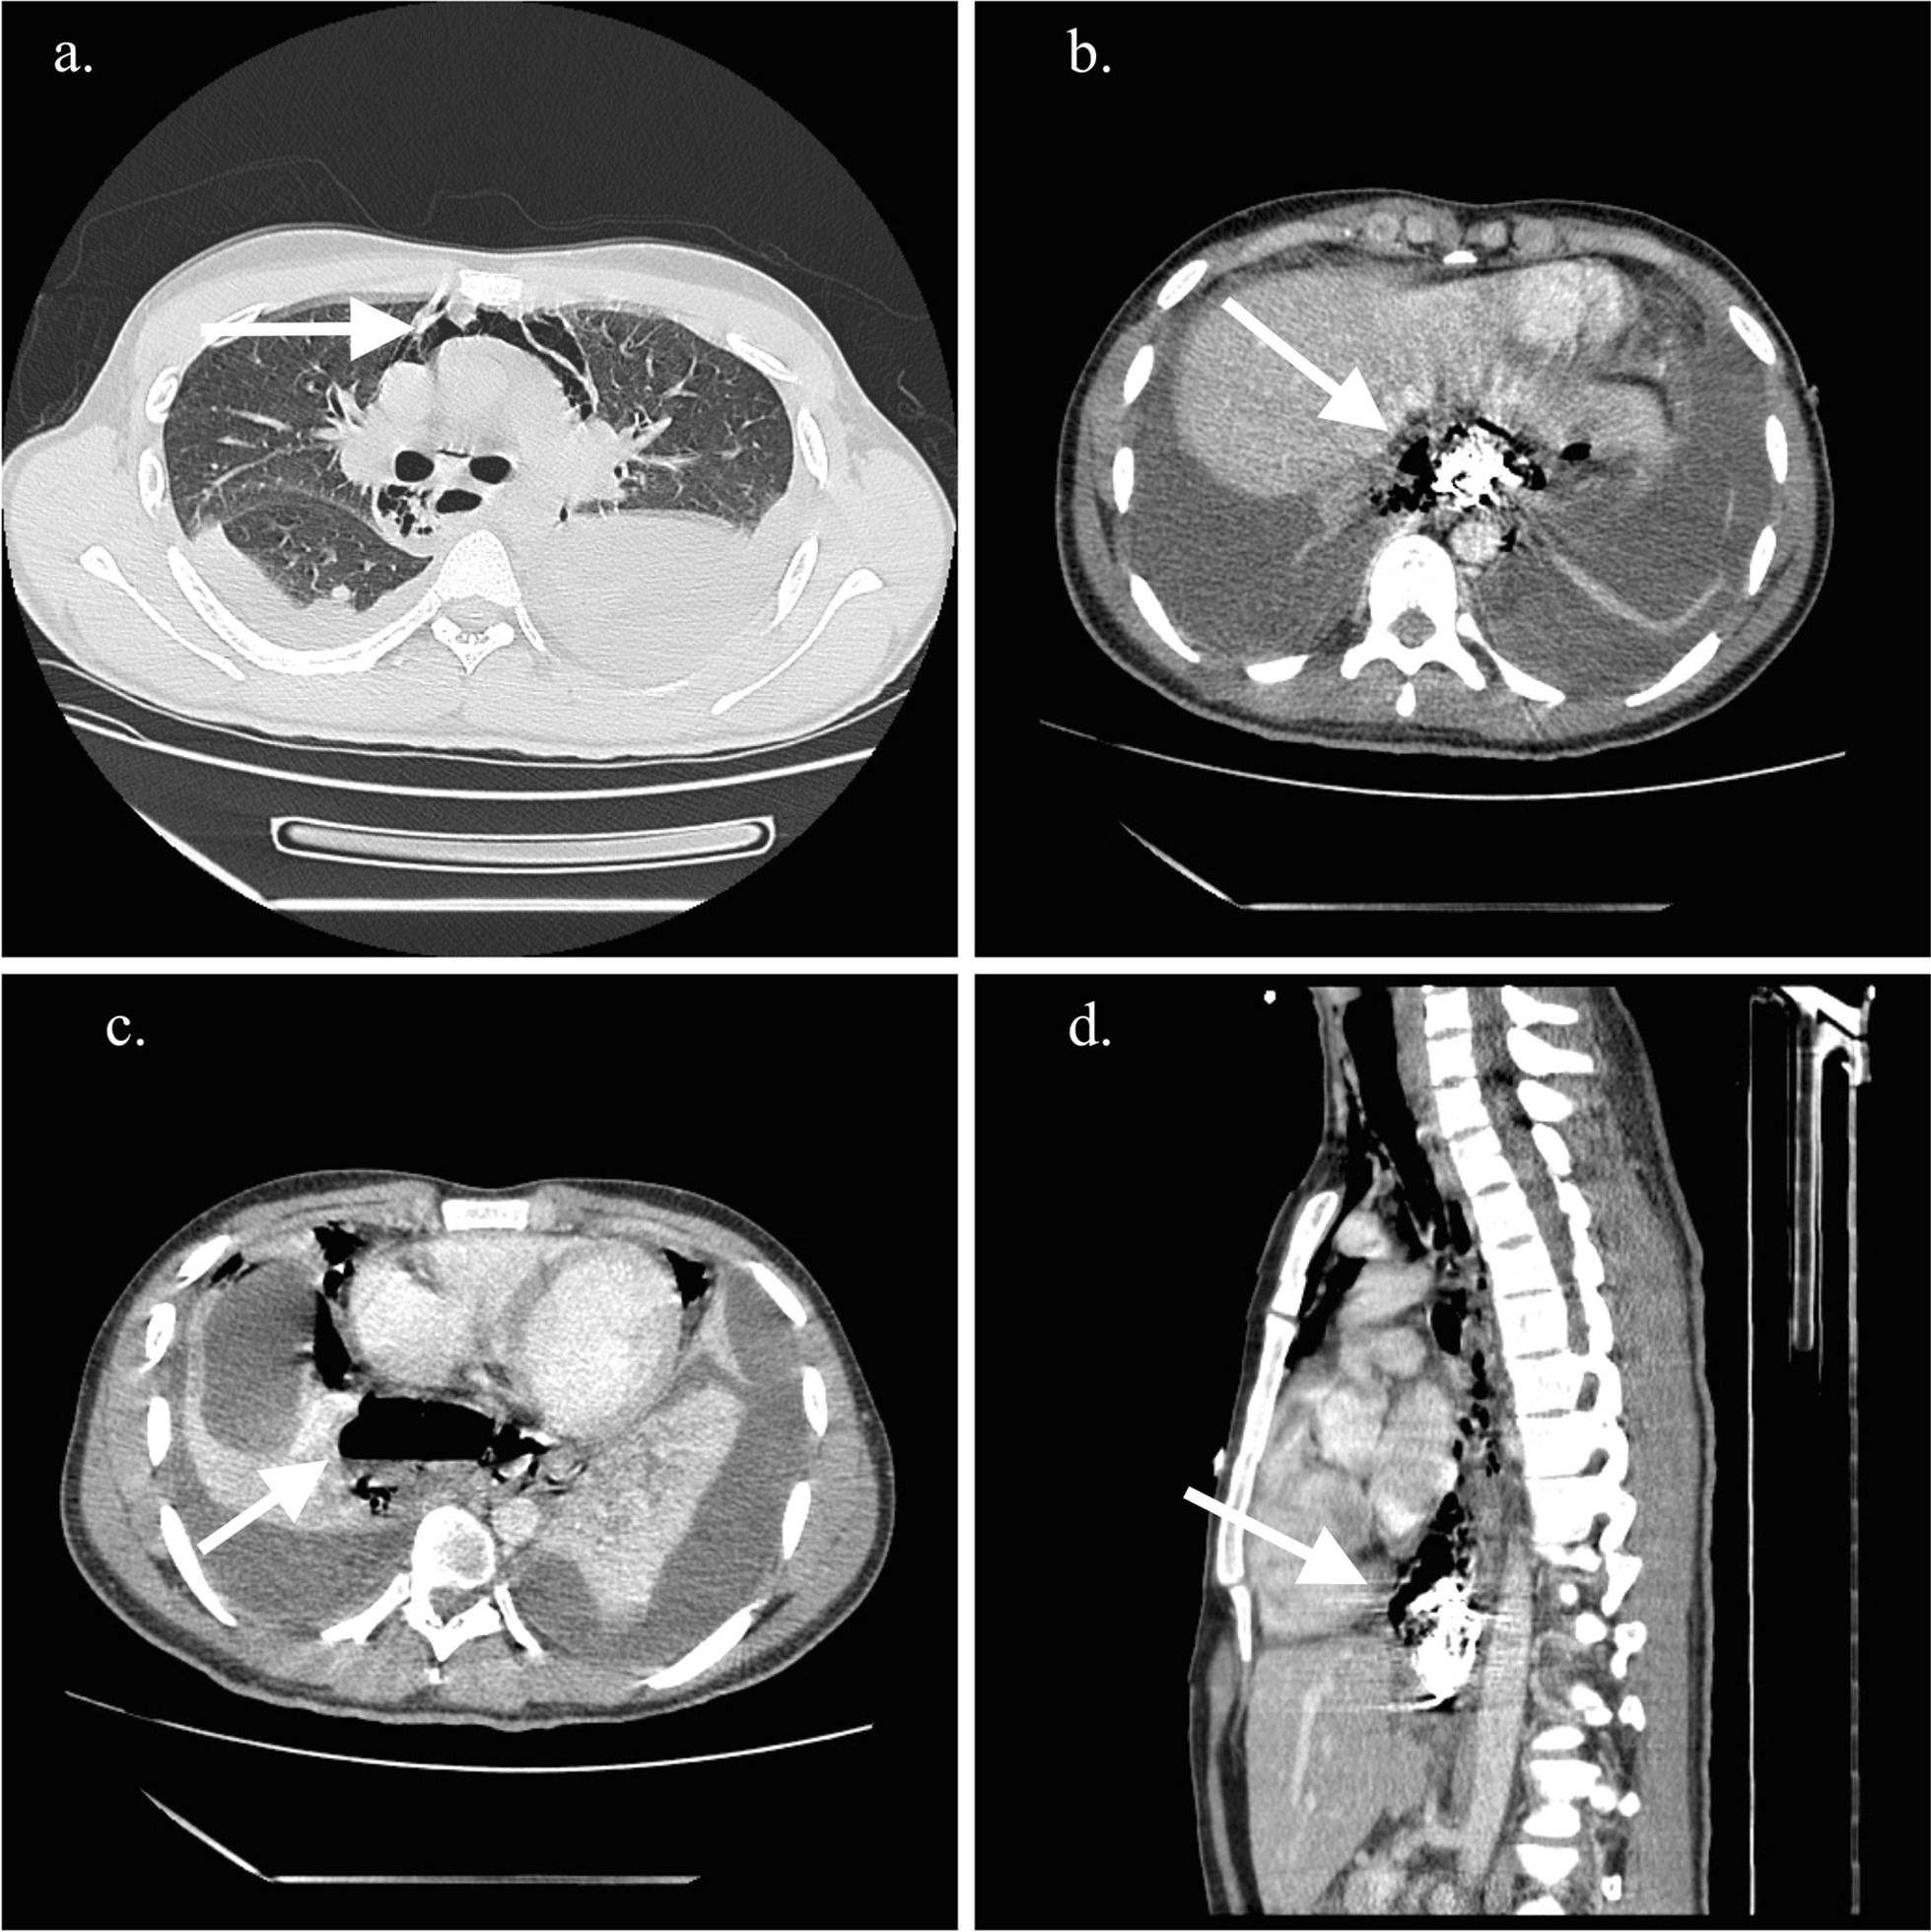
Contrast-enhanced CT findings. a CT findings of pneumomediastinum (white arrow), b extraluminal residual of oral contrast, c Fluid-air level in distal esophagus, d extraluminal oral contrast on perforation area (white arrow)
